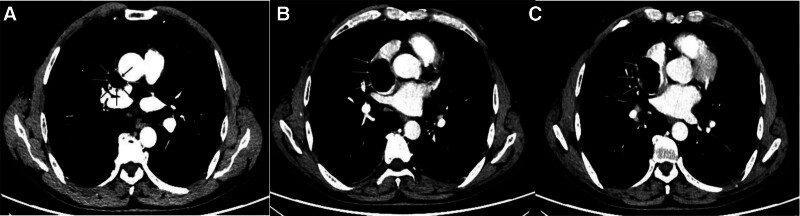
(A) A low-density mass is visible at the junction of the superior vena cava and the right atrium; (B) the mass has an intact capsule with clear boundaries, measuring approximately 4.1 × 2.8 cm, with a CT value of −91 HU; (C) There appears to be internal septation, and no enhancement is observed on enhanced scanning. CT = computed tomography.
